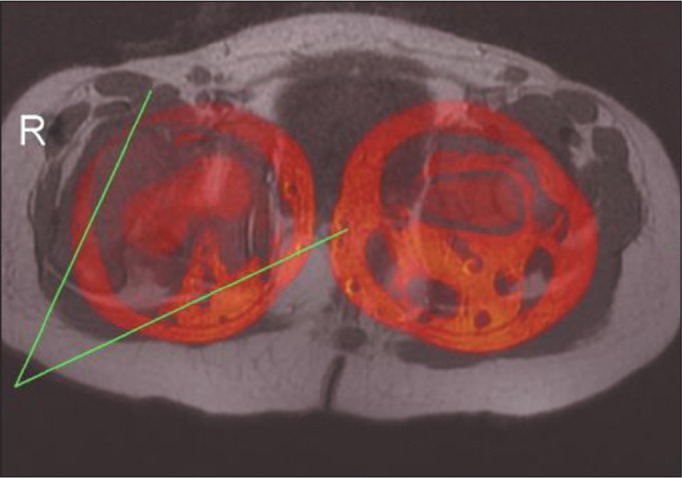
Fused axial T1-weighted FSE MRI sequence showing the lines employed
